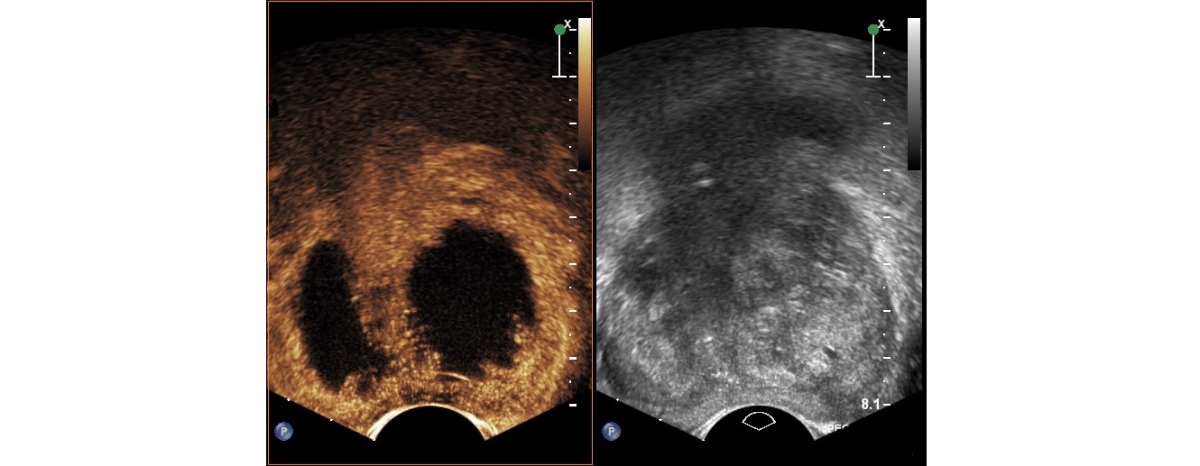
On the left the contract-enhanced ultrasound image of the prostate of the prostate 1 month following TPLA treatment, with dark areas corresponding with the treated areas. On the right the co-localized grey-scale image.
